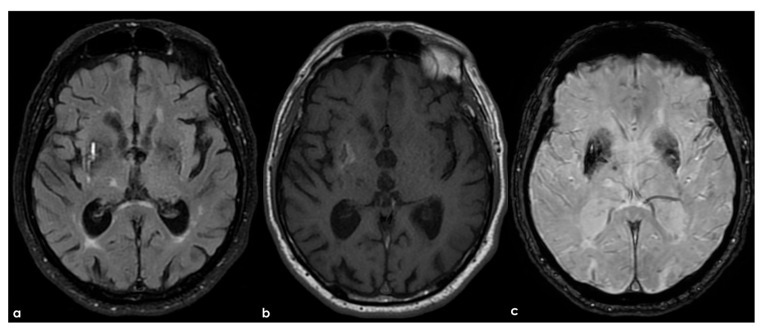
Brain MRI of a patient with an acute onset of a gait disorder and left hemisensory syndrome. The brain MRI was performed 2 months after symptom onset and the neurological examination found lower body parkinsonism. Panel (a) shows the axial fluid attenuated inversion recovery (FLAIR) sequence, panel (b) shows an axial T1W sequence, and panel (c) shows an axial susceptibility weighted sequence. A right (FLAIR hyperintense and T1W hypointense) hemorrhage occurred involving the lateral aspect of the putamen and the claustrum.
